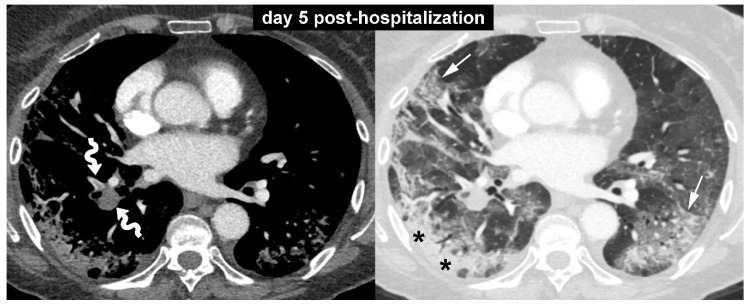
A 73-year-old woman was referred to our emergency department for the evaluation of a 10-day history of fever, dry cough, and shortness of breath. Her medical history was unremarkable except for a previous diagnosis of breast cancer. She had no history of lung comorbidities, such as asthma, chronic obstructive pulmonary disease, or interstitial lung disease. On presentation, the patient exhibited significant breathlessness, and arterial blood gas analysis revealed a pH of 7.51, PCO_2_ of 29 mmHg, PO_2_ of 43 mmHg, and oxygen saturation of 81%. Based on these data, 15 L/min of oxygen was administered through a non-re-breathing reservoir mask. Chest radiography showed bilateral interstitial and alveolar infiltrates in both lungs, greater on the right side. On the same day, the patient underwent nasopharyngeal swab, which confirmed the clinical suspicion of coronavirus disease (COVID-19). The following day the patient was transferred to an intermediate care unit. During the first few days in the hospital, her oxygen saturation was 90–95% with use of the non-re-breathing oxygen mask. On day five post-hospitalization, the patient experienced a sudden deterioration of lung function. Laboratory parameters also showed a significant increase in D-dimer levels (5250 ng/mL; reference value, less than 232 ng/mL). Based on these data, contrast-enhanced computed tomography (CT) scan was performed. The contrast-enhanced CT images with mediastinal and lung window setting (Figure 1) showed filling defects in some branches of the pulmonary artery (curved arrows) and bilateral ground-glass opacities (arrows) associated with areas of consolidation (asterisks). No evidence of pulmonary fibrosis was found in this initial CT examination.
